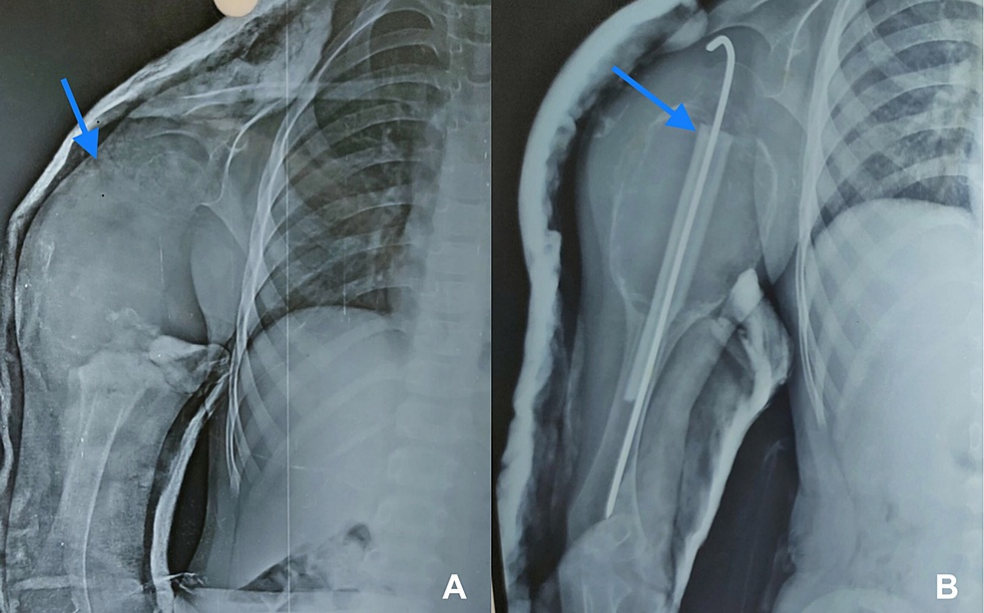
Preoperative (A) and postoperative (B) radiograph (anteroposterior view) showing curettage of the lesion with bone grafting and fixation with K-wire. K-wire: Kirschner wire
